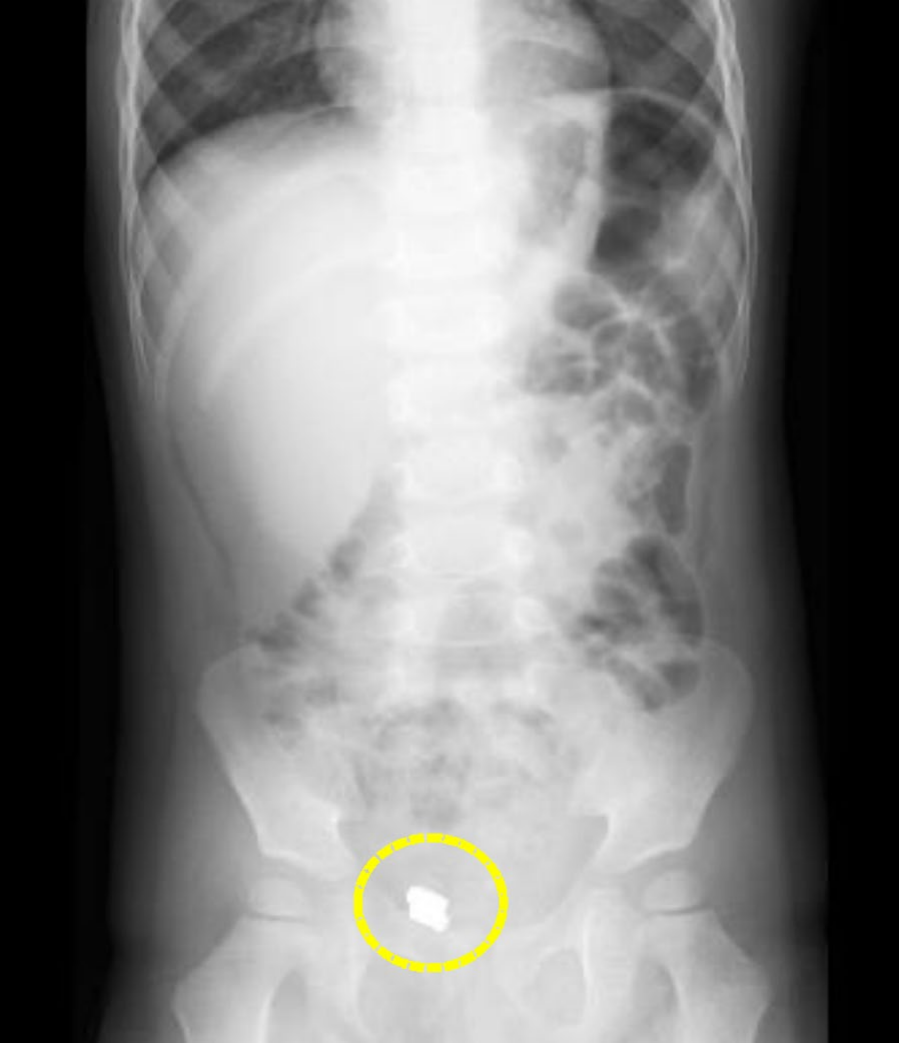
X-ray imaging. X-ray image revealed the presence of a 1.0-cm radiopaque FB (yellow circle) located in the right lower abdomen area on day 1. We expected that bowel movement might be able to evacuate the FB
